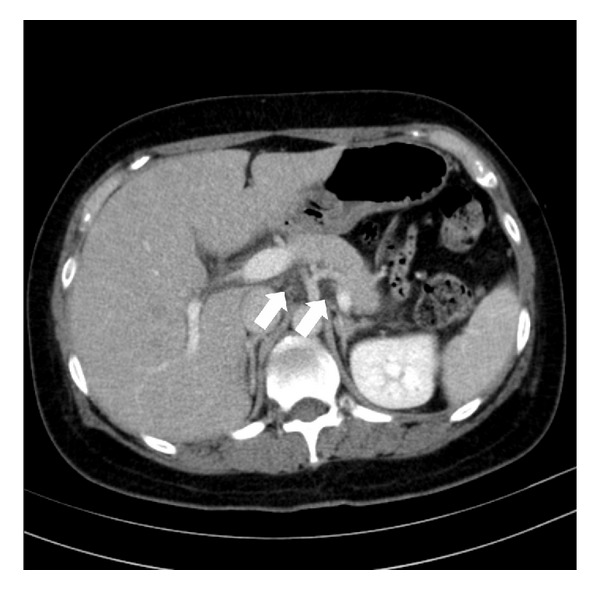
Abdominal computed tomography (CT) scan taken 24 h after admission. The image shows a mild enlargement of the pancreas and a diffuse inflammation of the peripancreatic tissues (⇒); however, there was no exudate. There was no pancreatic necrosis. The CT severity index is 2.
